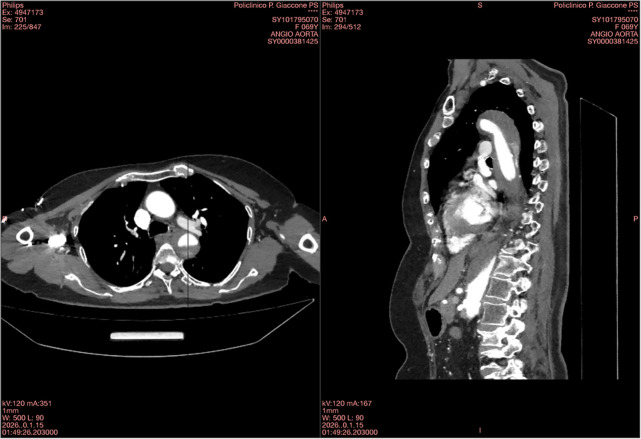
CT angiography on day 7 showing IMH progression with early signs of type B aortic dissection.
